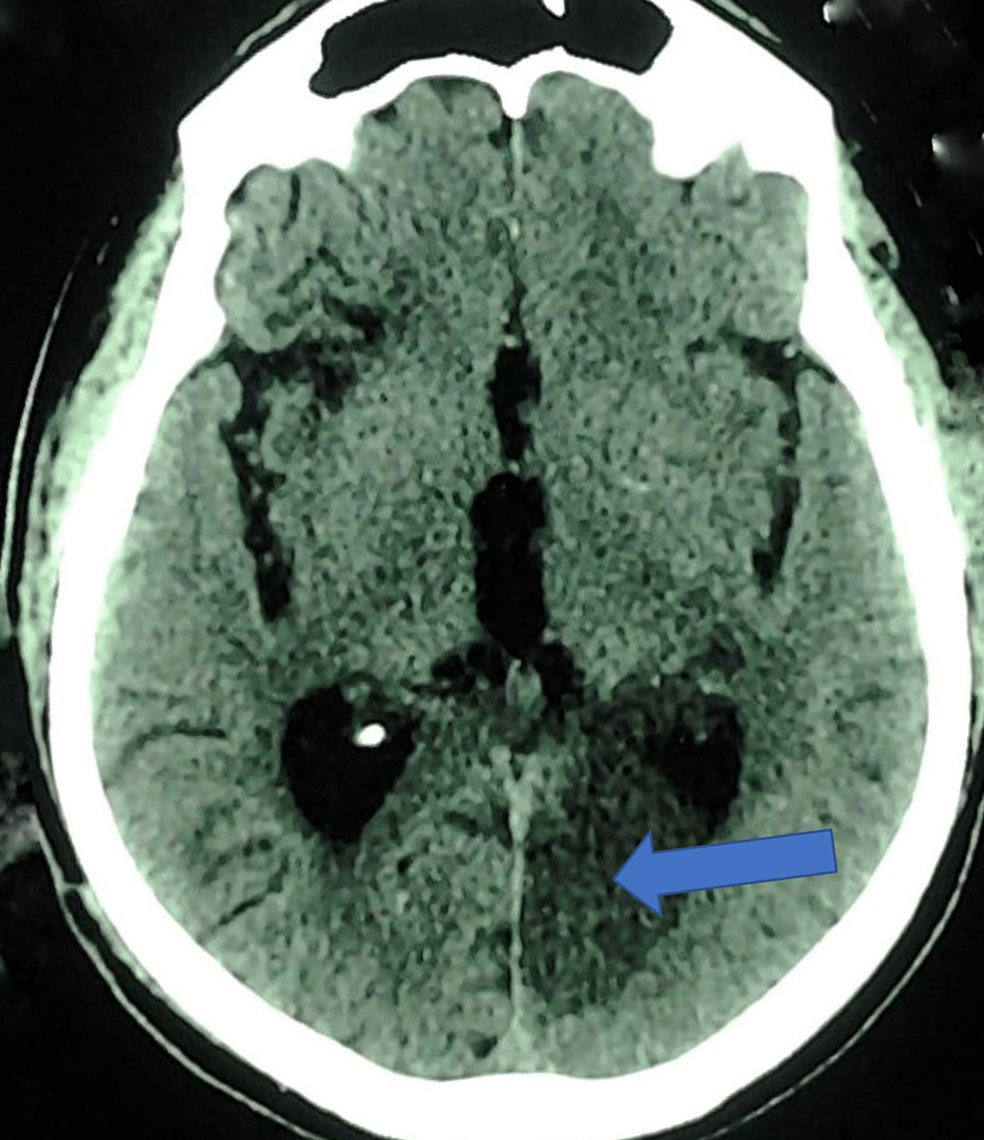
Plain CT axial section in Case 2 showing hypodensity in left medial occipital region with mild mass effect to occipital horn of left lateral ventricle (blue arrow). No hyperdensity within to suggest hemorrhagic transformation; bilateral peri ventricular lucency seen.
